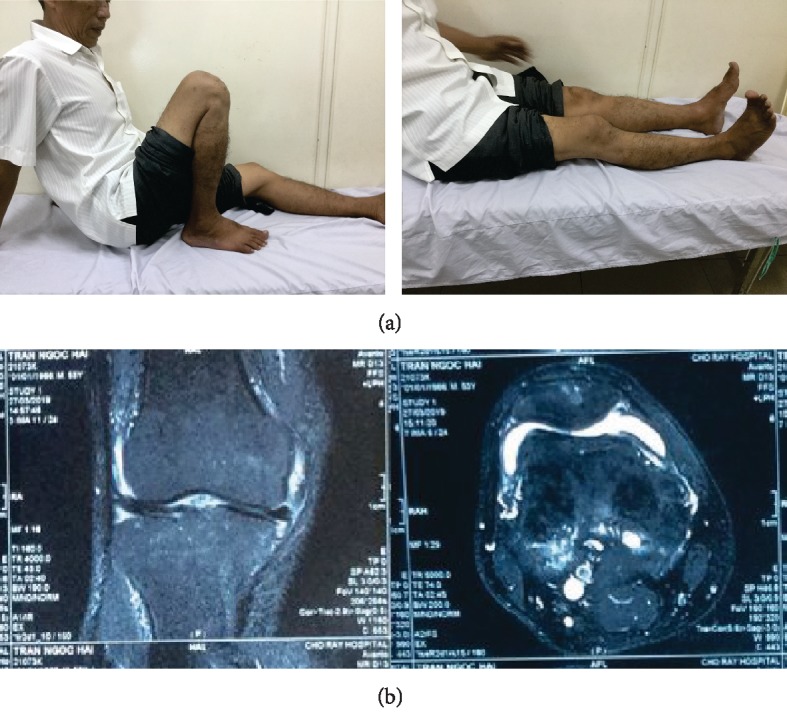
(a) The last follow-up, more than 1 year. (b) MRI of the last follow-up.
